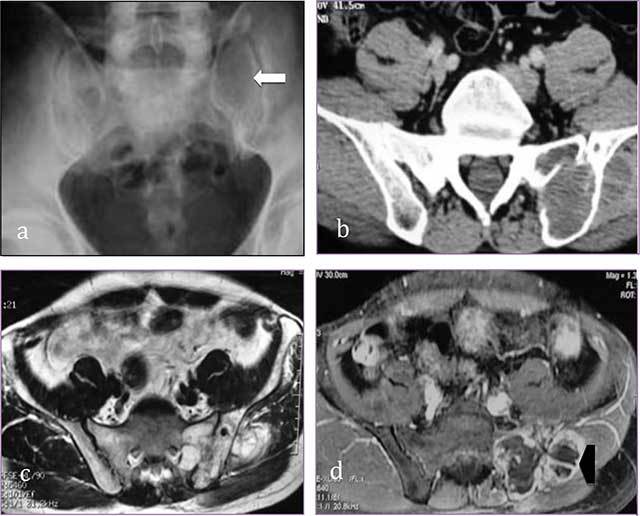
Sacral chondrosarcoma. (a) Plain radiograph of pelvis shows an ill-defined osteolytic lesion of left sacrum (white arrow). (b) transversal CT scan shows extension through the left sacroiliac joint, the ilium and the gluteal muscles. (c, d) MR: transversal T2-WI, transversal T1-WI after gadolinium contrast injection show a lobulated mass predominantly of high signal intensity on T2-WI with ring-and-arc enhancement (arrowhead).
